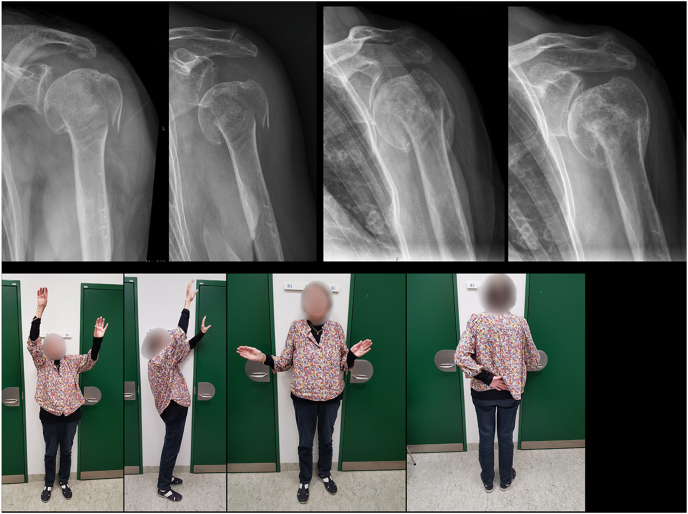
A 90-year-old female with a centrally impacted surgical neck fracture. During healing, the humeral shaft became further impacted. Although the fracture healed with shortening, the patient achieved pain-free shoulder function. The humeral head became better centered in the glenoid during rehabilitation and as healing progressed. External rotation remained restricted. Early signs of glenohumeral osteoarthritis were present. Anterior–posterior radiographs were taken at admission, 2 weeks, 6 weeks, and at 6 months. Photographs were taken at 6 months.
